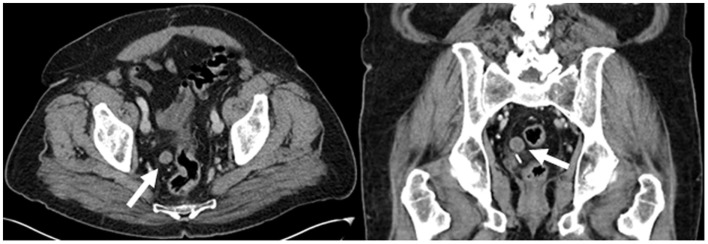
Contrast-enhanced CT demonstrates a well circumscribed round soft tissue density measuring 1.7 cm in the right hemipelvis adjacent to the sigmoid colon (white arrows).
